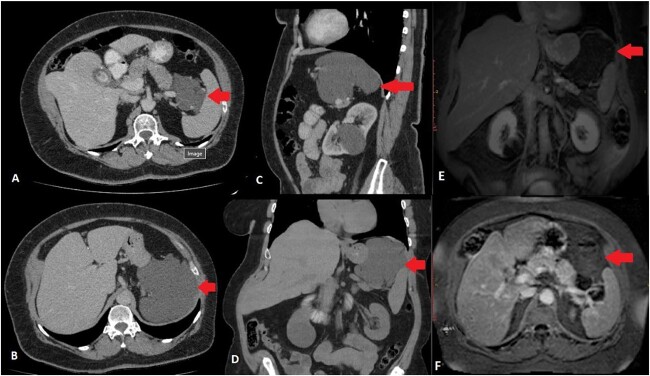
CT scan of the abdomen. Transverse scan—mass between pancreas and spleen (A), Transverse scan—mass expanding below the diaphragm (B), Sagittal scan—mass below the diaphragm and over the tail of the pancreas and splenic vessels (C), Coronal scan—mass between the diaphragm, upper pole of the spleen and tail of the pancreas (D), MRI scan of the abdomen. Coronal scan—mass between the diaphragm, upper pole of the spleen and tail of the pancreas, not enhancing (E), Transverse scan—mass between pylorus, pancreas, and spleen (F) ( arrow marking the mass).
